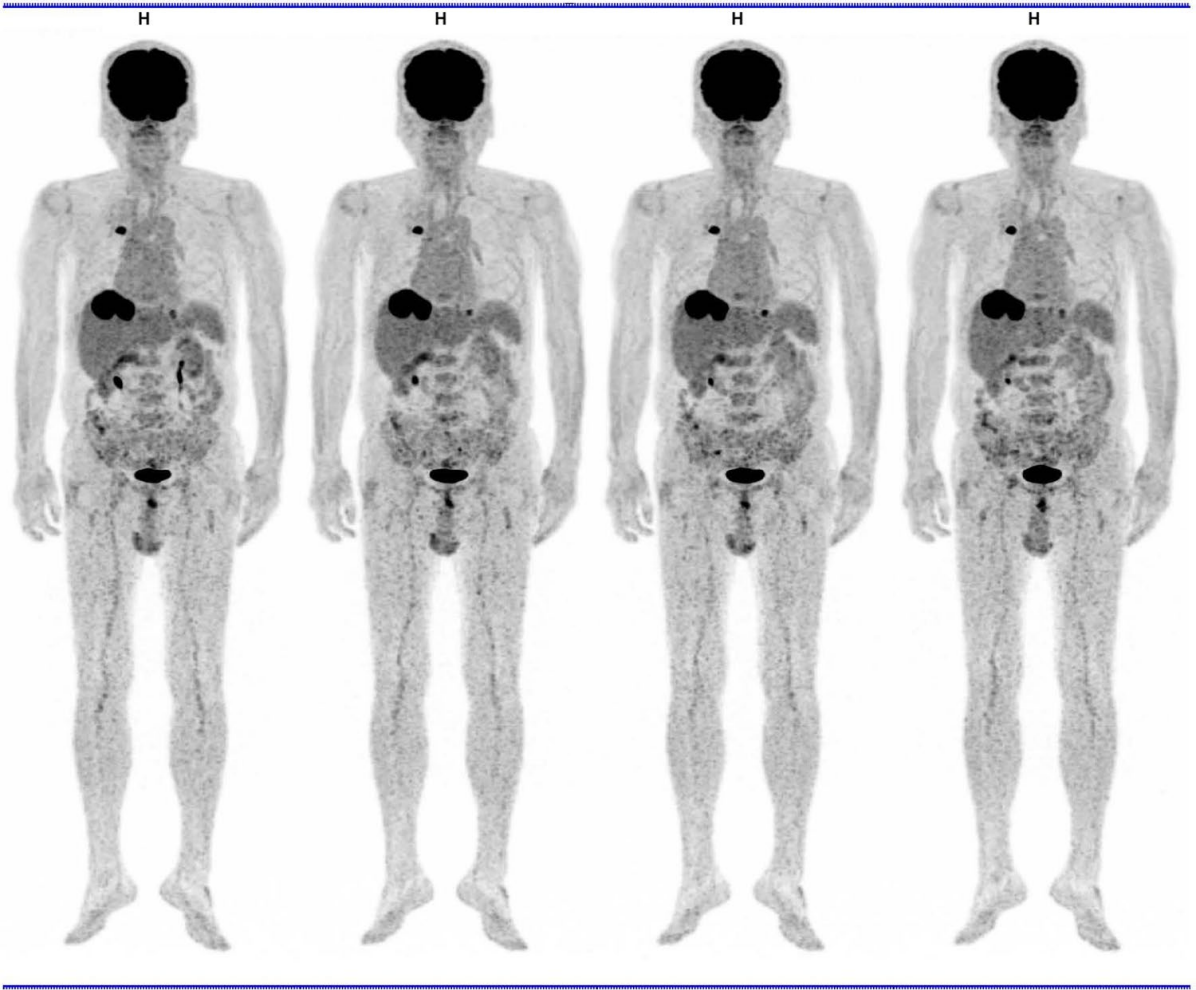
Serial whole-body FDG-PET images (3 min each) approximately 60 min after FDG administration in a patient with lung cancer with pulmonary metastatic lung cancers. High and persistent uptake in the lung cancer and liver metastasis was noted, whereas some uptake motion changes were observed in the ureter and small intestine
